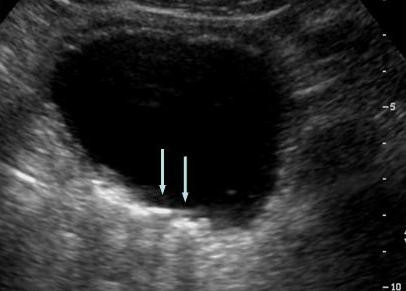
Abdominal ultrasound. Note the linear calcifications in the posterior wall of urinary bladder with posterior acoustic shadowing (arrows).
